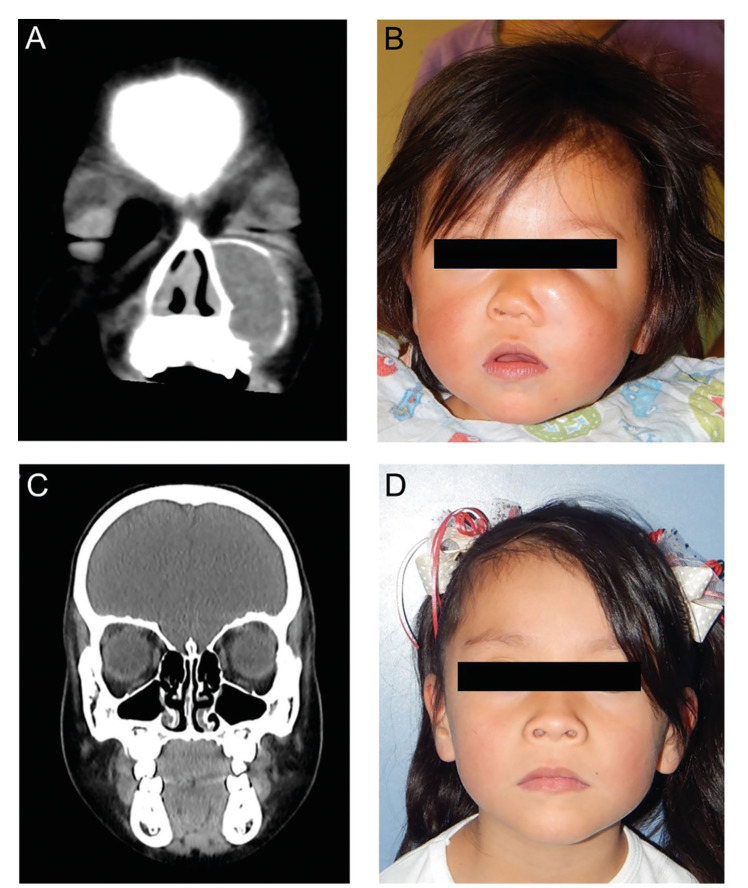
Intraosseous myofibroma of the maxilla (case 13). A) Pre-surgical computed tomography scan of 1-year-old female patient. Note the hypodensity of the lesion delimited by bone sclerosis; B) Pre-surgical facial photograph (increase volume corresponding to three months of evolution); C) Computed tomography scan after 4 years of surgical excision; D) Facial photograph after 4 years of treatment.
